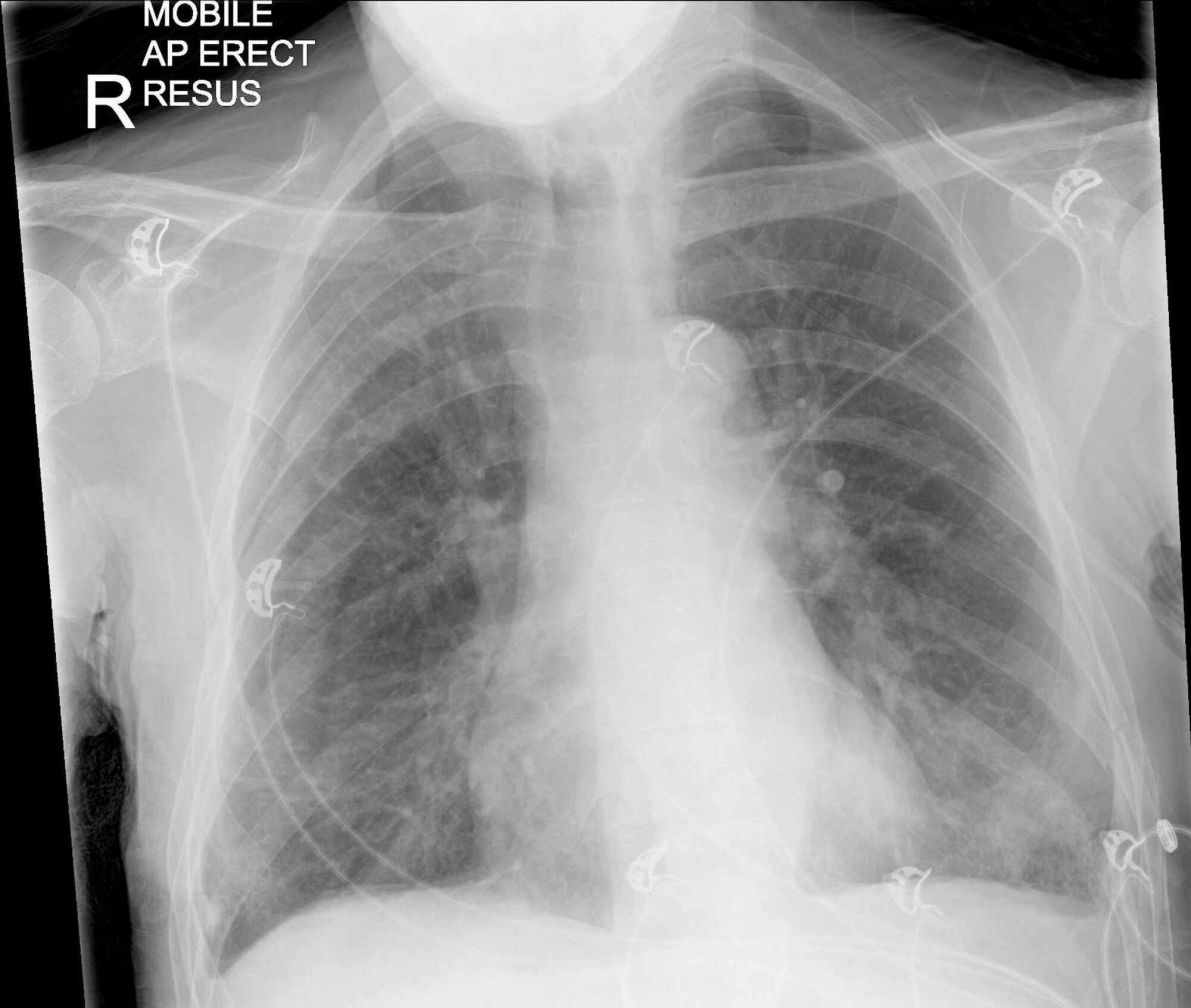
Chest radiograph taken on arrival to accident and emergency Radiograph shows lung hyperinflation, consistent with chronic obstructive airways disease, and diffuse peripheral infiltrates suggestive of viral pneumonia.
